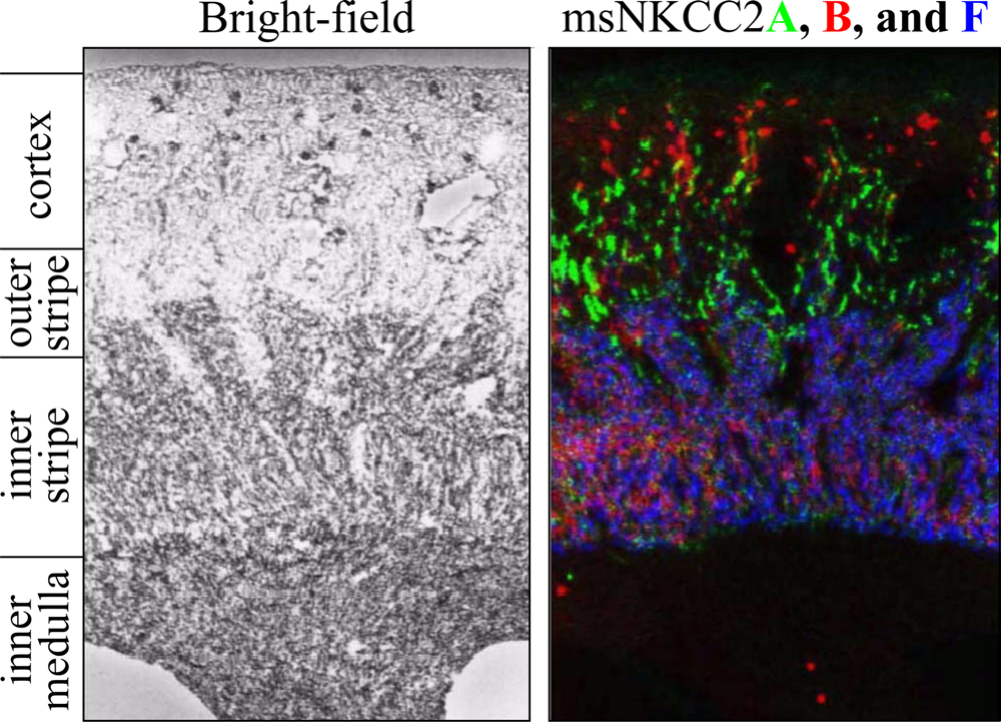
Distribution of Na^+^–K^+^–Cl^−^ cotransporter 2 (NKCC2) splice variants in adult ms kidney by in situ hybridization. Exon 4 was detected for each variant with a specific radioactive riboprobe on a sagittal tissue section, signals were converted to colors (red for NKCC2B, green for NKCC2A, and blue for NKCC2F) and images were superimposed. Adapted with permission from Igarashi et al. (1995). The color mount was generated by Dr Biff Forbush (Yale University, CT)
